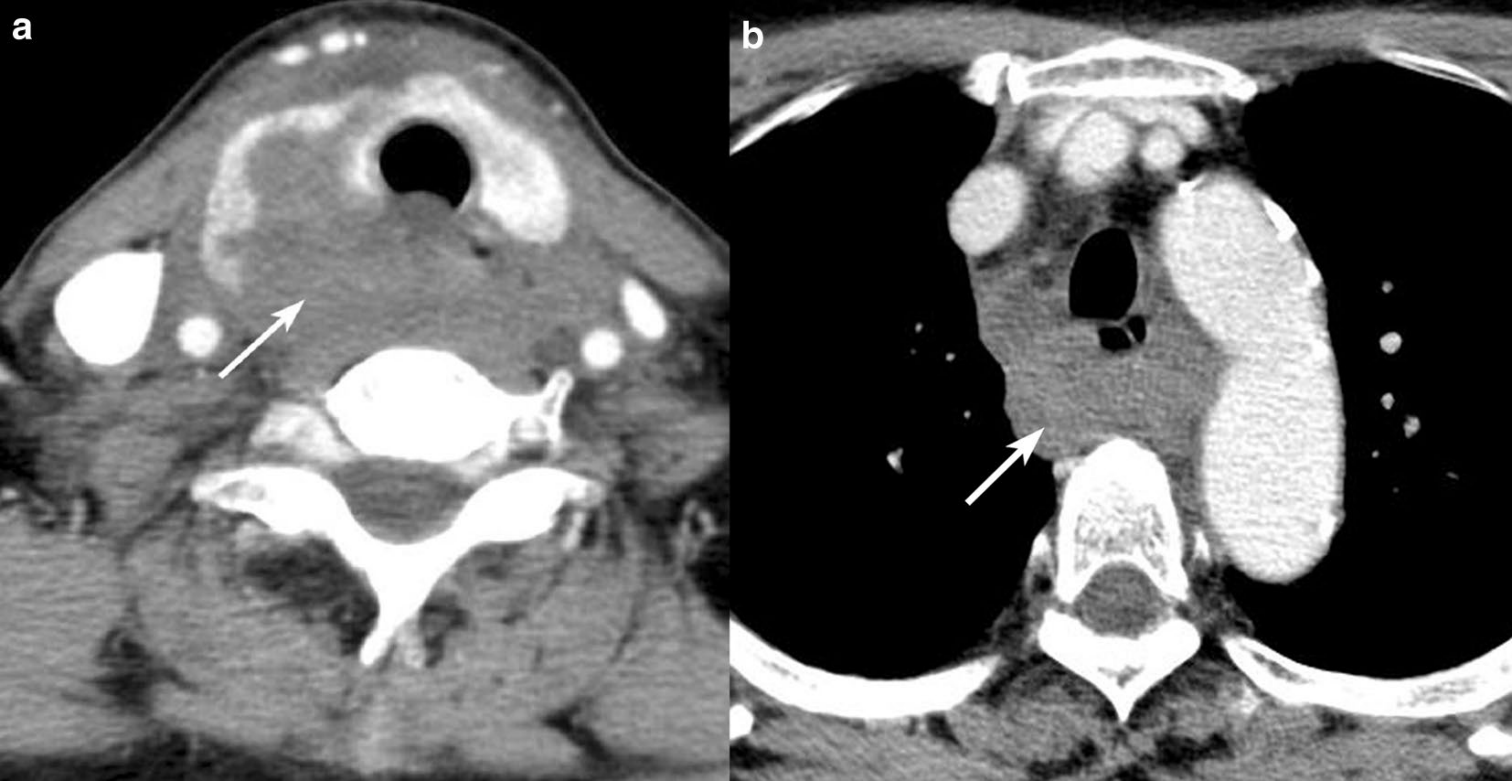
Computed tomography of the neck and chest. Computed tomography showing thyroid rupture, with a large hematoma (*arrow*) extending from the neck (a) to the mediastinum (b)
